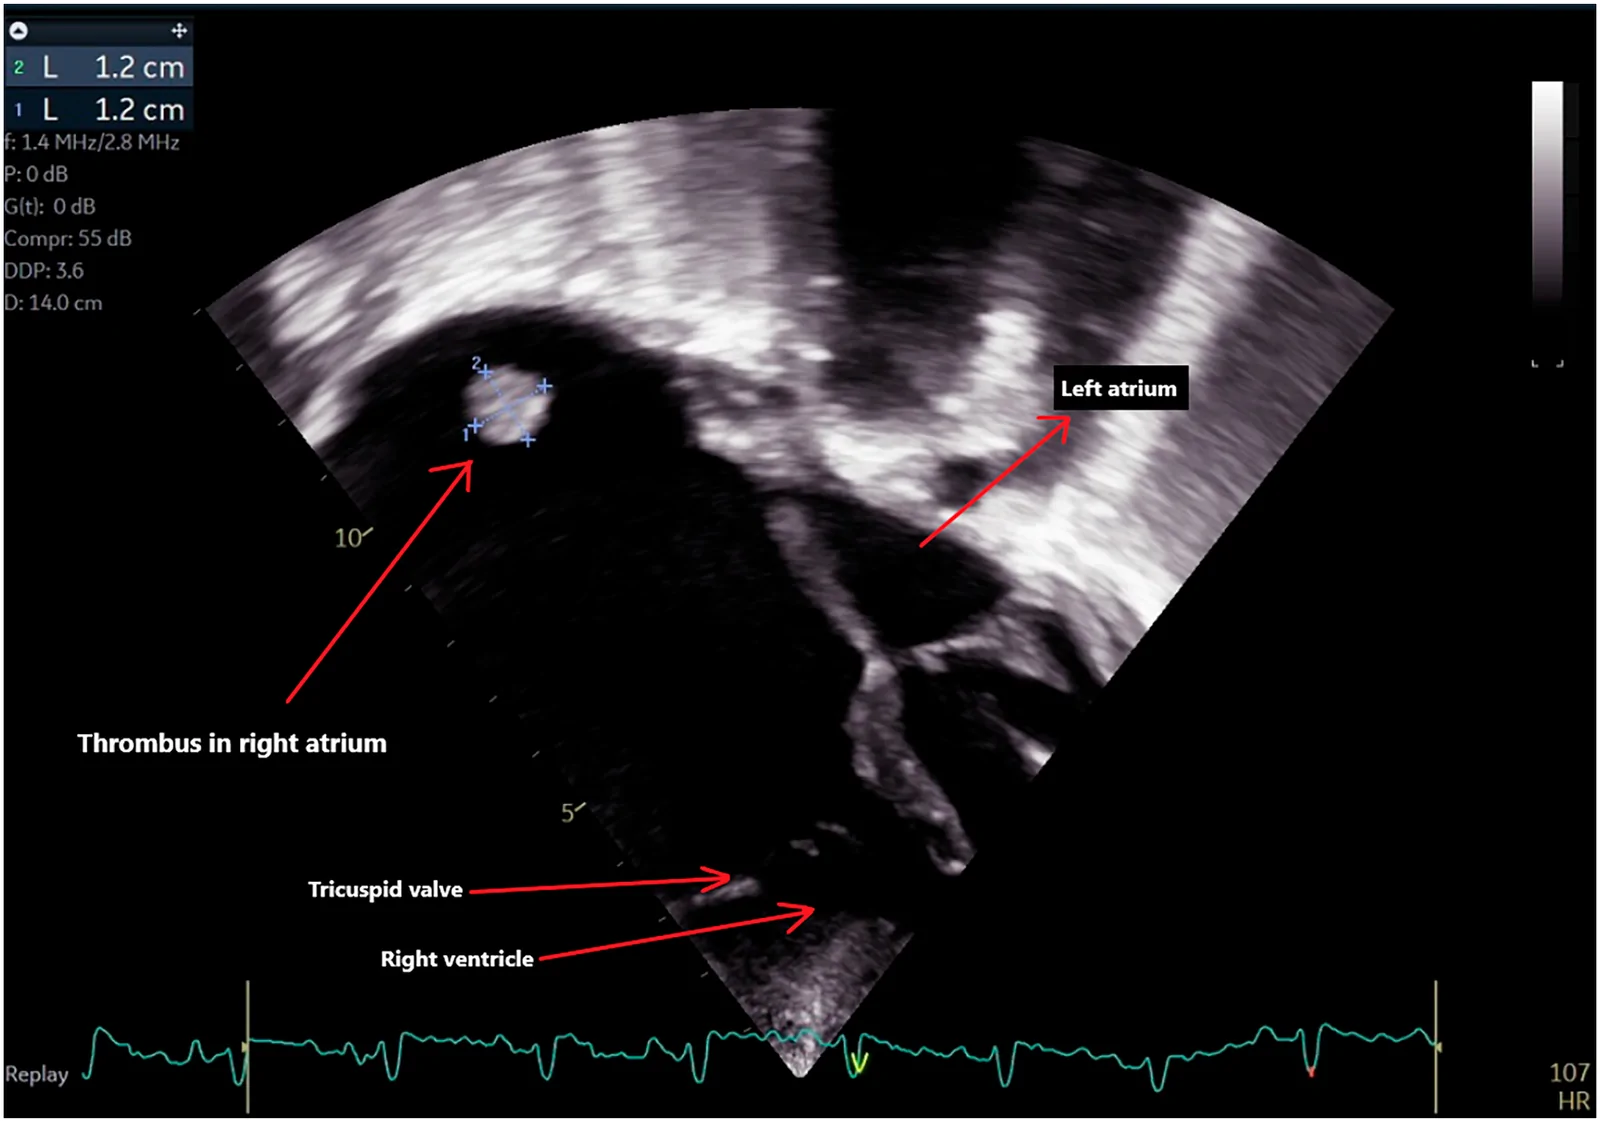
Echocardiography Image Demonstrating Thrombus in the Dilated Right Atrium, Measuring 1.2 × 1.2 cm
